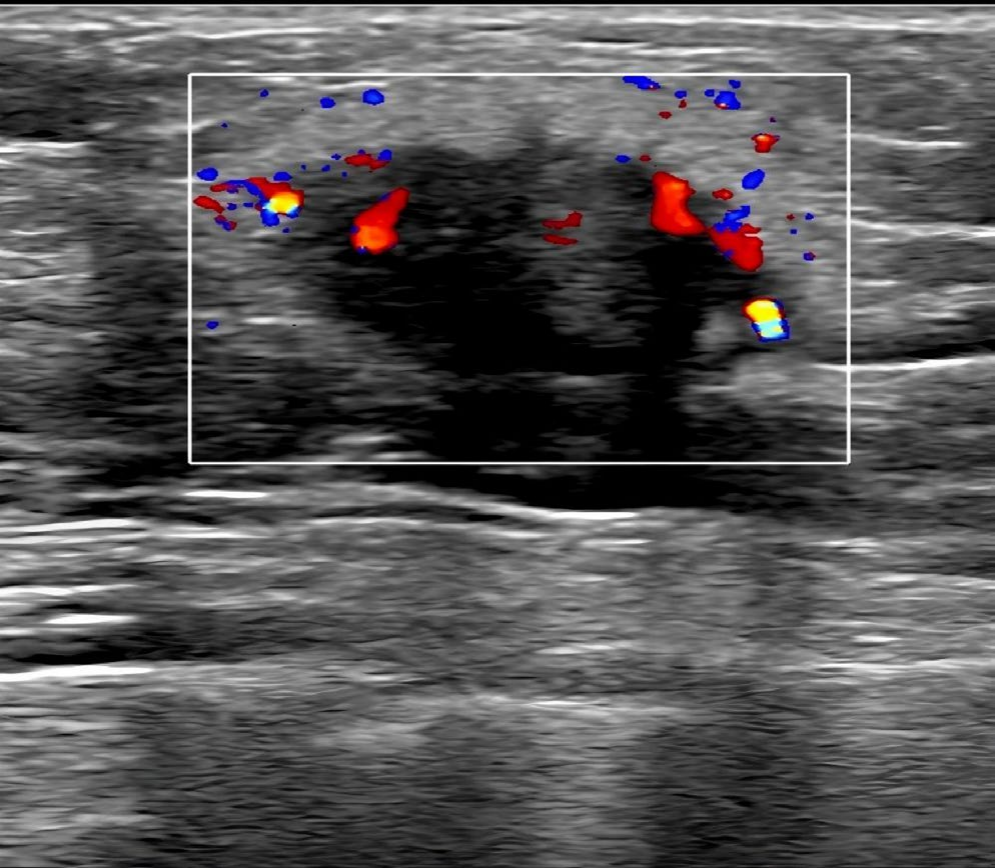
Breast ultrasonography showing a hypoechoic nodule measuring 13 × 13 × 8 mm with clear boundaries, irregular shape, and inhomogeneous internal echo located beneath the left nipple.
